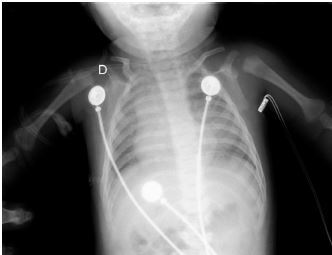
Portable anteroposterior chest radiograph showing no focal infiltrate and limited due to patient rotation.
